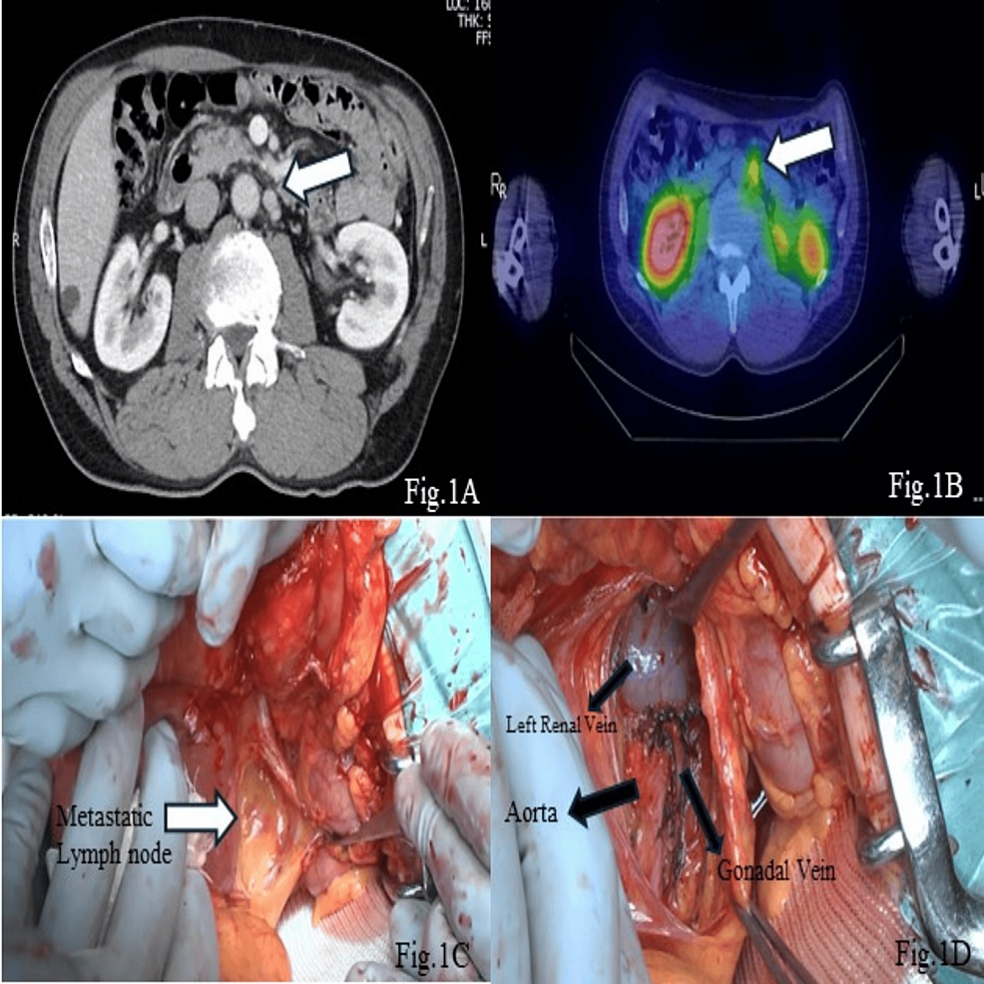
Preoperative images of the metastatic lymph node and anatomical location of the metastatic lymph node. Figure 1A: CT image of the mass (shown with white arrow). Figure 1B: Somatostatin receptor scintigraphy image of the mass (shown with white arrow). Figure 1C: Metastatic lymph node in the para-aortic station (shown with white arrow). Figure 1D: Gonadal vein, left renal vein, and aorta visible after resection of the metastatic lymph node.
